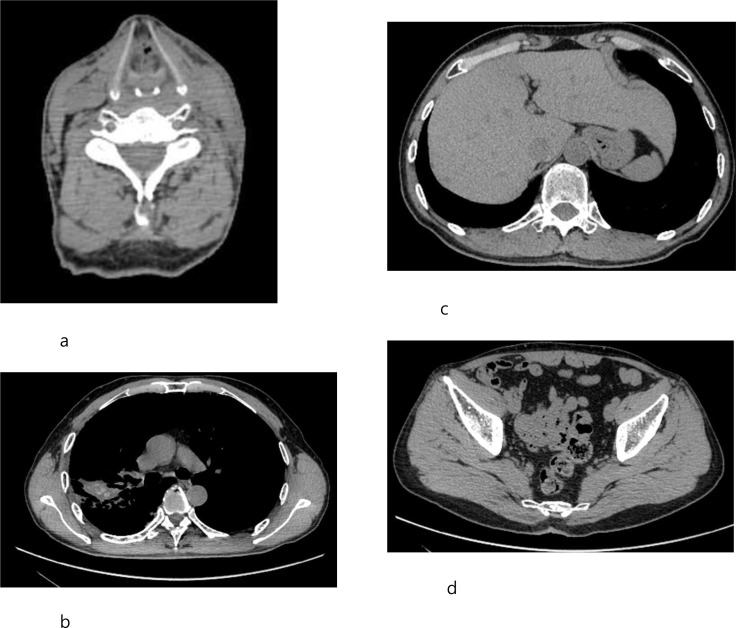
March 2026 CT follow-up scan. (a) The original thyroid lesion shows no progression. (b) The original lung lesion shows no progression. (c) The original liver lesion shows no progression. (d) The original pelvic lesion shows no progression.
